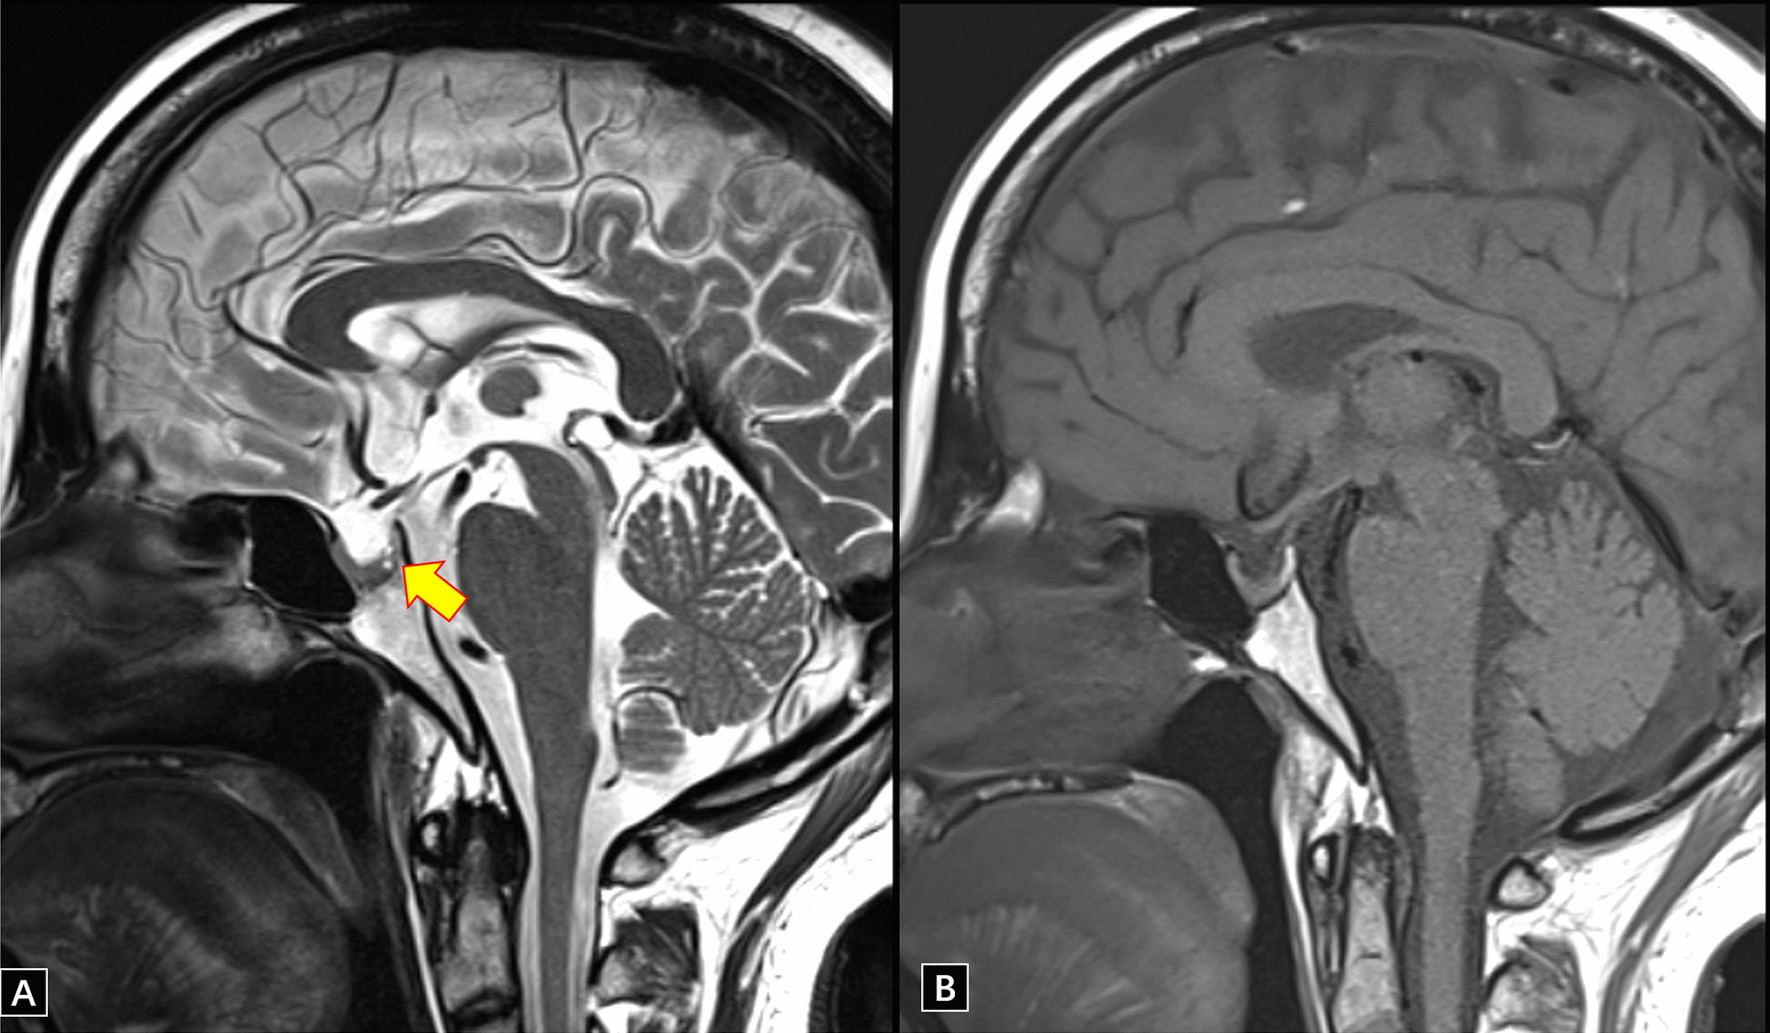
The patient’s pituitary MRI on August 2, 2021. A is a T2WI sagittal image, showing two nodular T2 hyperintensity shadows on the back of the pituitary gland as indicated by the arrow, and B is T1WI with no obvious lesions
